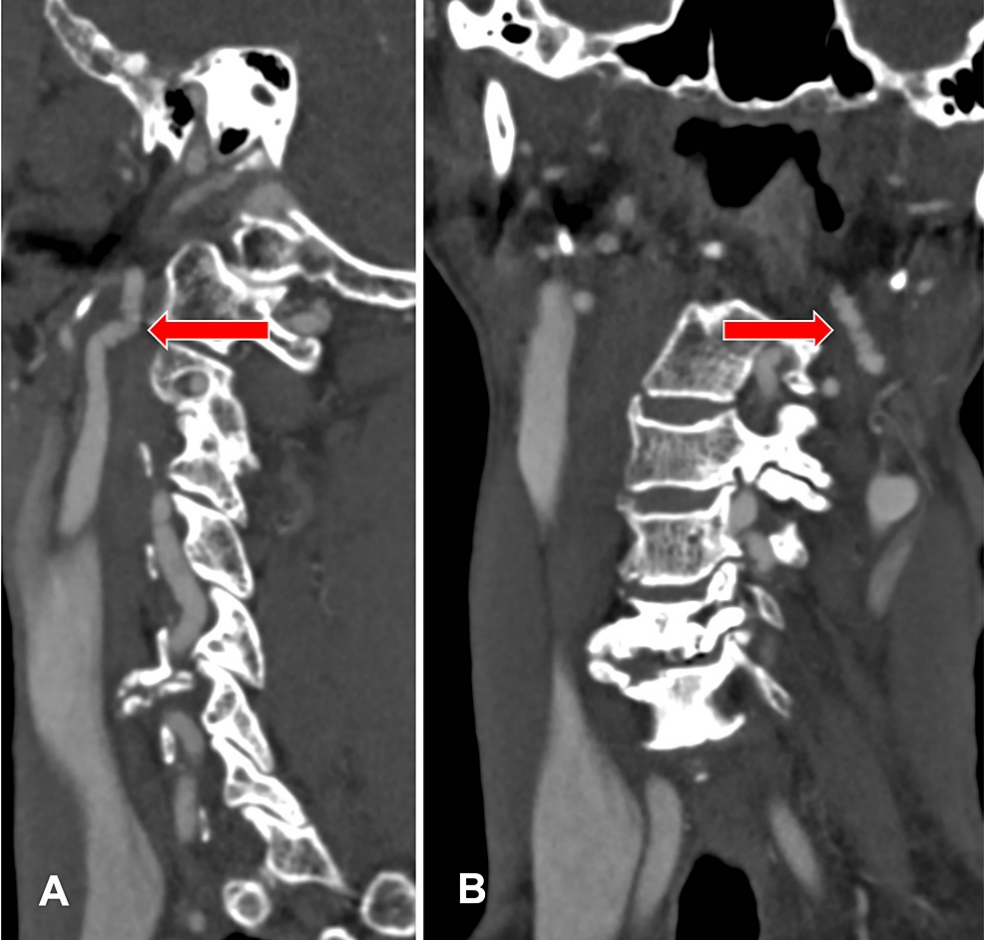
Sagittal CTA of the right internal carotid artery Sagittal computed tomography angiography (CTA) of the right internal carotid artery (A) and coronal CTA of the left internal carotid artery (B) shows alternating mild stenosis and dilatation, causing a string of beads appearance bilaterally (red arrow).
